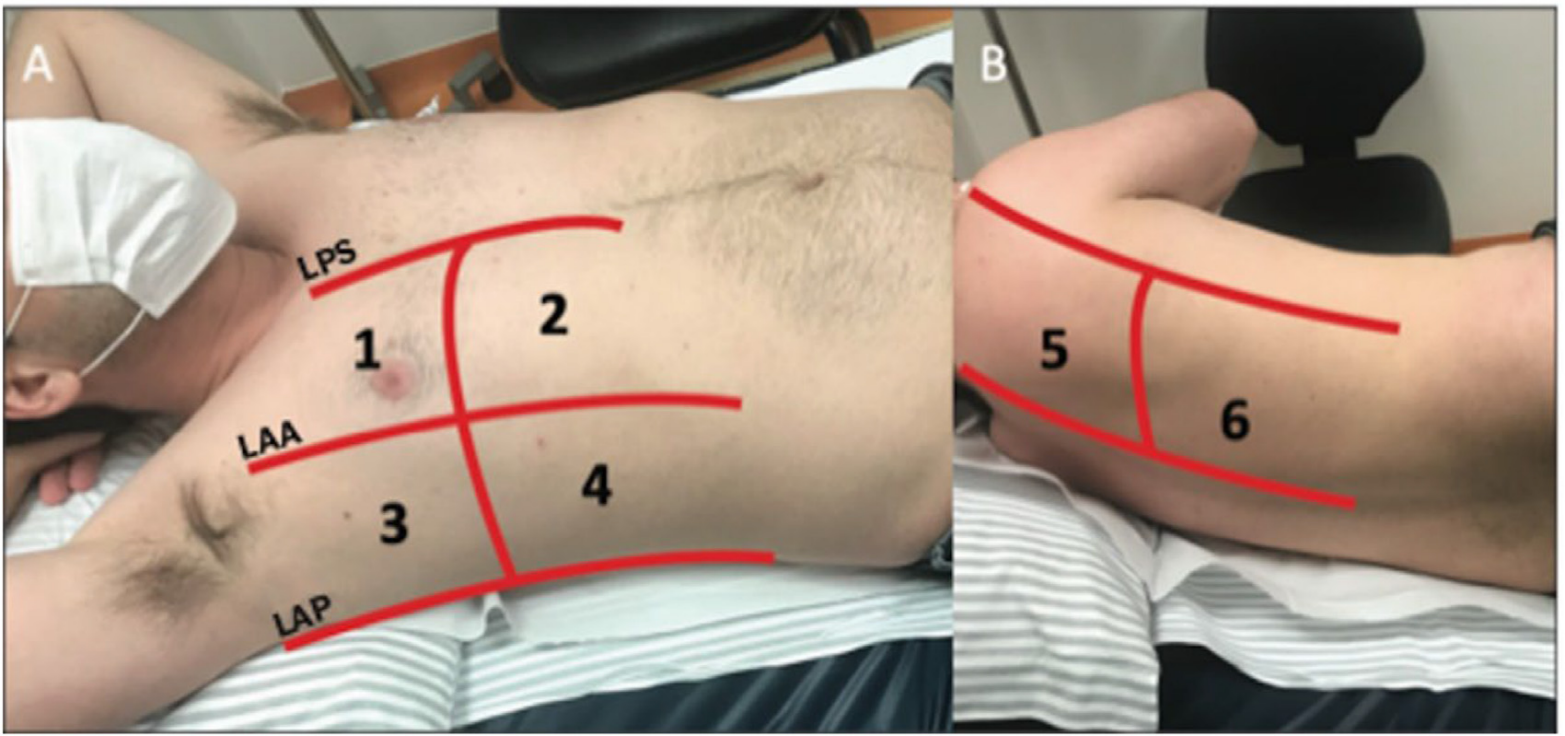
Ultrasound zones of the chest (taken from Oliveira RR, Rodrigues TP, Savoia P, Gomes AC, Chammas MC. Lung ultrasound: an additional tool in COVID-19. Radiol Bras.); (A) Horizontal supine position. (B) Left lateral decubitus. LPS, Parasternal Line; LAA, Anterior Axillary Line; LAP, Posterior Axillary Line. 1, Anterosuperior region; 2, Anteroinferior region; 3, Upper lateral region; 4, Lower lateral region; 5, Posterosuperior region; 6, Posteroinferior region.
